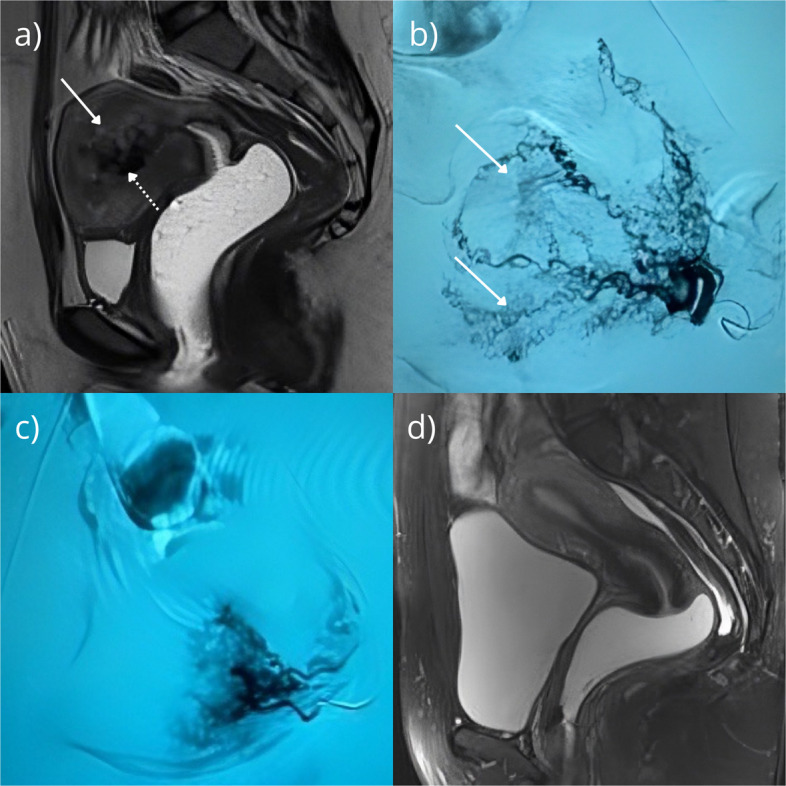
a Sagittal T2-weighted MRI demonstrating serpiginous flow-related signal voids (dashed arrow) with associated T2-hyperintense vascular lakes (solid arrow). b Left uterine artery angiogram shows dilated and spiraling intrauterine branches with apparent disorganization persistent in the uterine left lateral wall, in addition to blush areas (solid arrow); no nidus detected. c Left uterine artery angiogram post-embolization of 500–700 µm microspheres. Note the other intrauterine branches that were initially less opacified and later without filling opacification. d T2 Fat Sat 6 months after UAE shows no evidence of residual lesion and preservation of uterine morphology (myometrial and endometrial)
